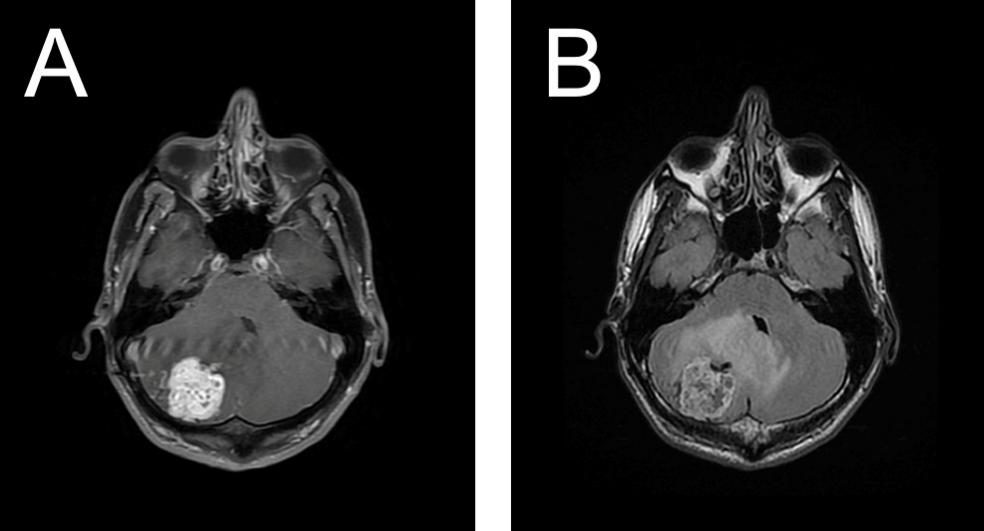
Pre-operative MRI shows a right cerebellar mass with associated vasogenic edema. (A) Axial T1-weighted contrast-enhanced image demonstrates avidly enhancing mass in the right posterior cerebellar hemisphere abutting the pial surface, with mass effect causing partial effacement of the fourth ventricle, consistent with hemangioblastoma. (B) Axial T2-FLAIR weighted image demonstrates a heterogenous, intraparenchymal mass in the right posterior cerebellar hemisphere abutting the pial surface, with perilesional cysts and intratumoral flow voids. There is marked vasogenic edema with partial effacement of the fourth ventricle.
